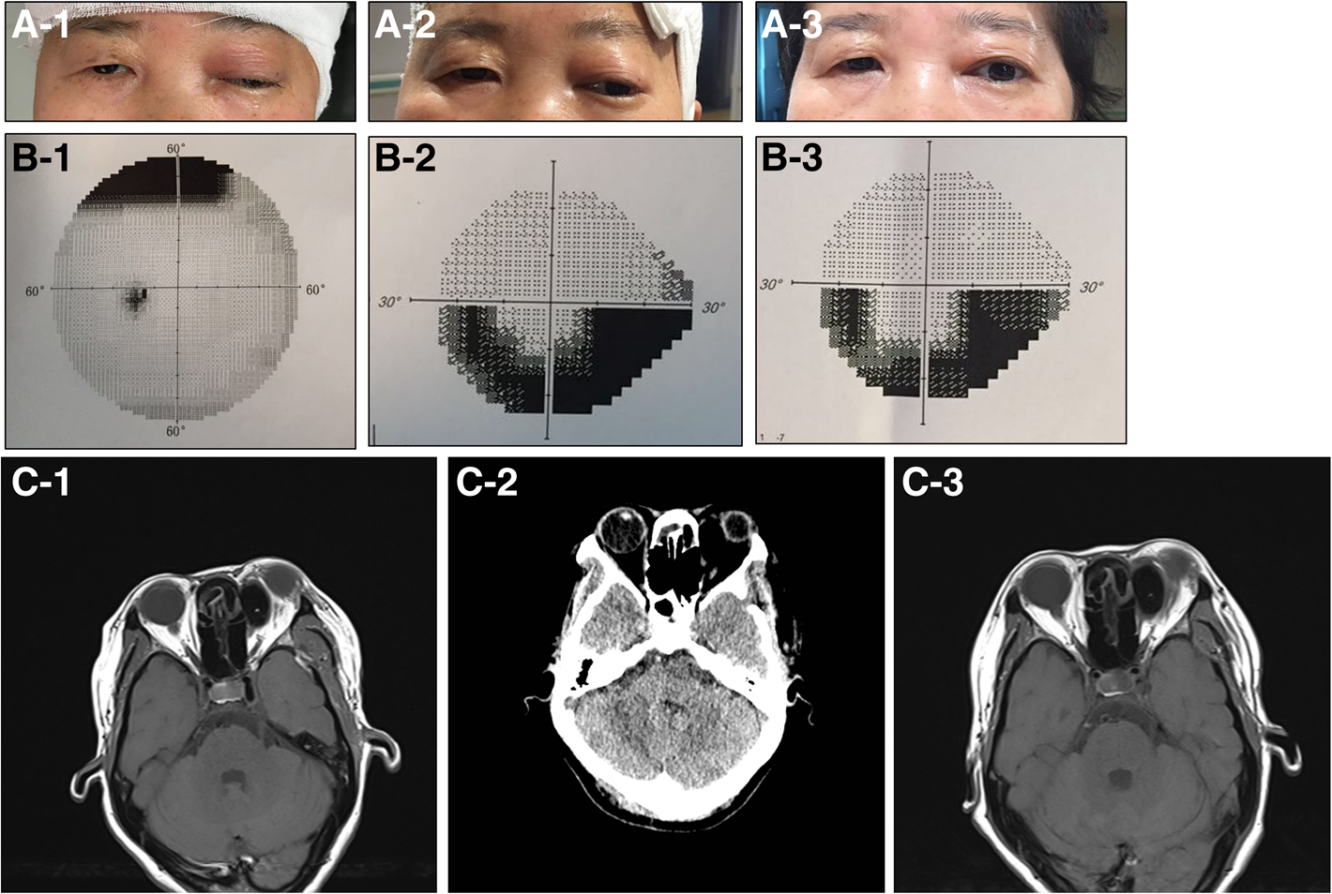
a: The ptosis and protosis of the left eye. A-1: POD 3; A-2: POD 1mo.; A-3: POD 6mo. b: The visual field of the left eye. B-1: POD 3; B-2: POD 1mo.; B-3: POD 6mo. c: MRI/CT of the orbit. C-1: POD 3; C-2: POD 1mo.; (CT); C-3: POD 6mo
